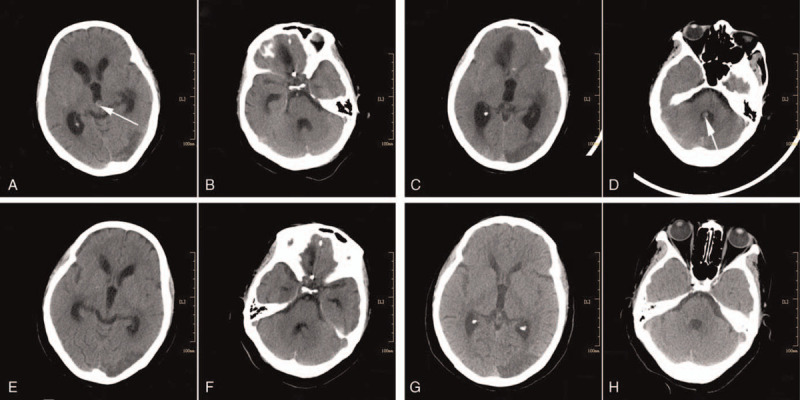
(A and B) Computed tomography (CT) performed at 10:13 am on day 19 showed that there was a blood clot in the third ventricle (3 V, as indicated by the arrow), accompanied by mild hydrocephalus. No clot was seen in the fourth ventricle (4 V). (C and D) CT performed at 2:57 pm on day 19 showed hydrocephalus aggravation, and the previous clot located currently in the 4 V. (E and F) CT performed on day 21 showed remarkable improvement of the hydrocephalus and complete dissolution of the clot in the 4 V. (G and H) At follow-up 2 months after discharge, CT revealed nonrecurrence of hydrocephalus.
